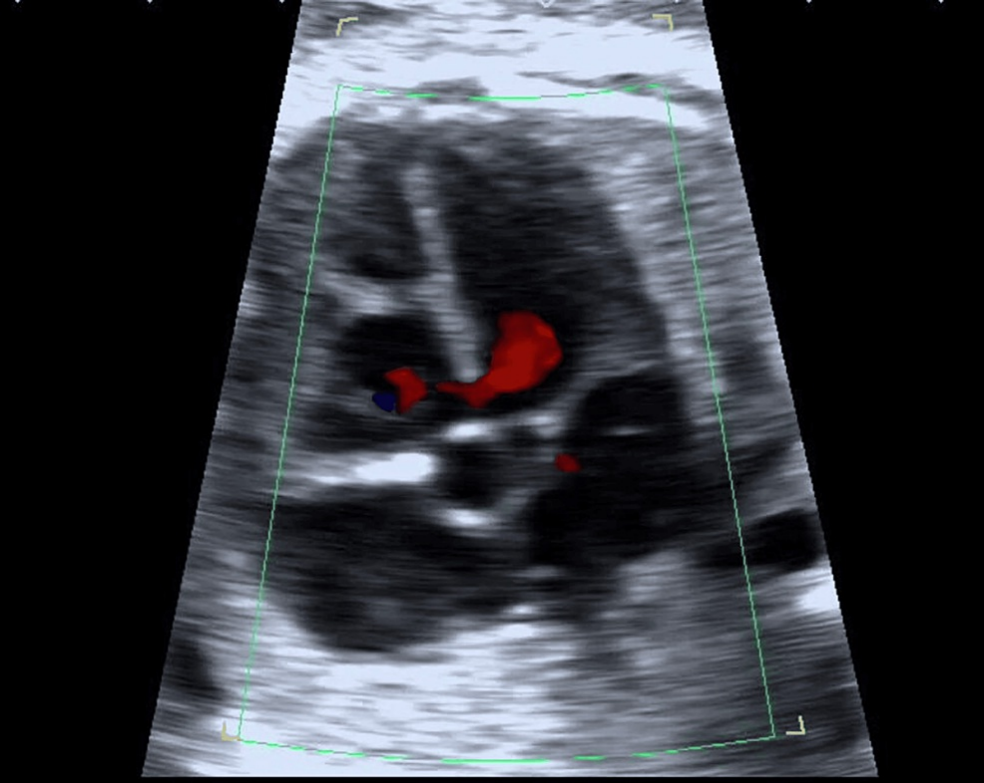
Echocardiogram showing ventricular septal defect (VSD)
